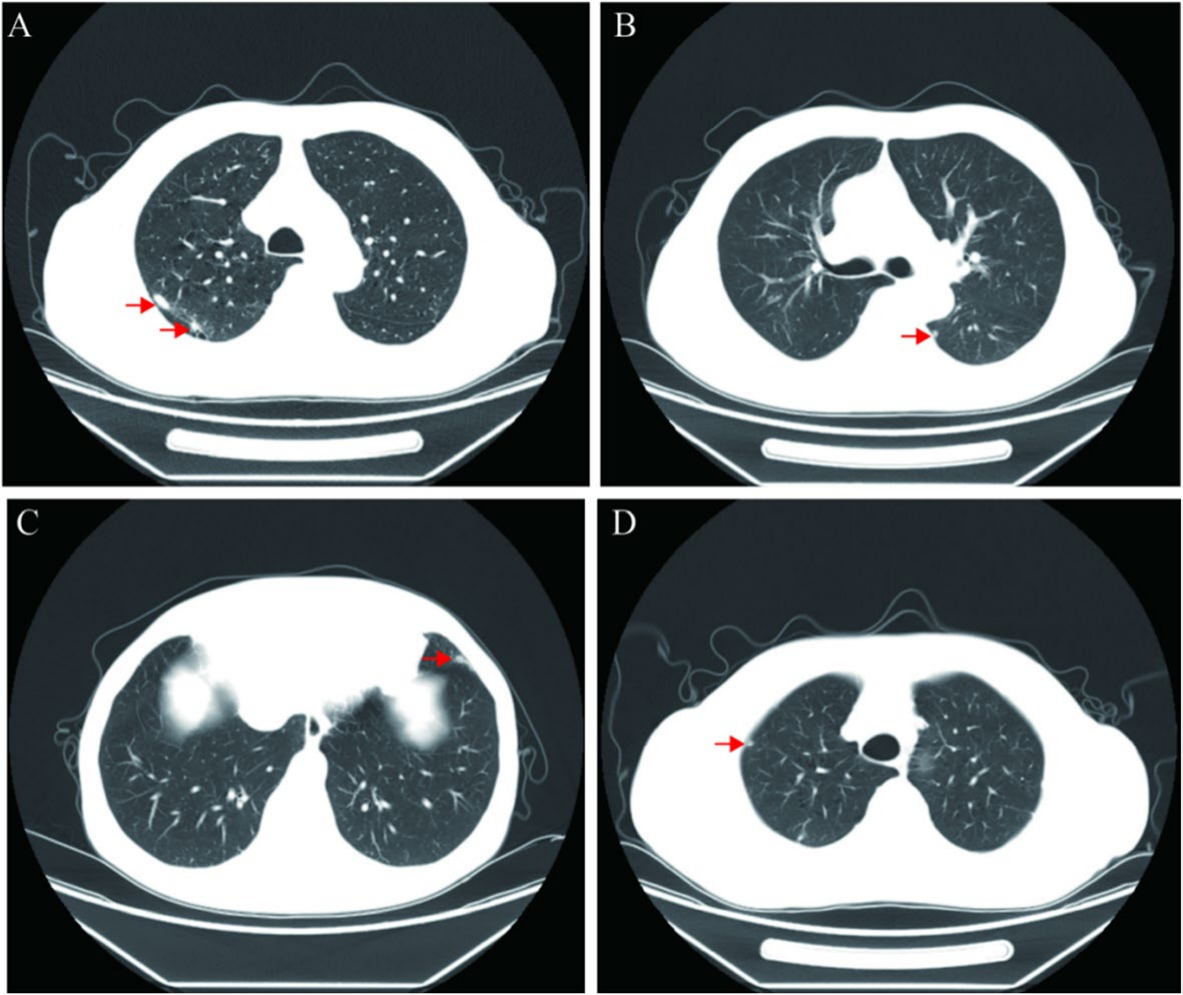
Enhanced computed tomography (CT) results of lung lobe: A 5.0 × 4.0 × 1.0 cm nodule was detected in the post-apical segment of the left upper lobe,1.5 × 1.0 × 1.0 cm nodule was detected in theapical segment of the left upper lobe; B-DThree tiny lung nodules(benign nodule with a measured diameter of 0.2–0.9 cm) detected in the lung and other parts of the right lung
